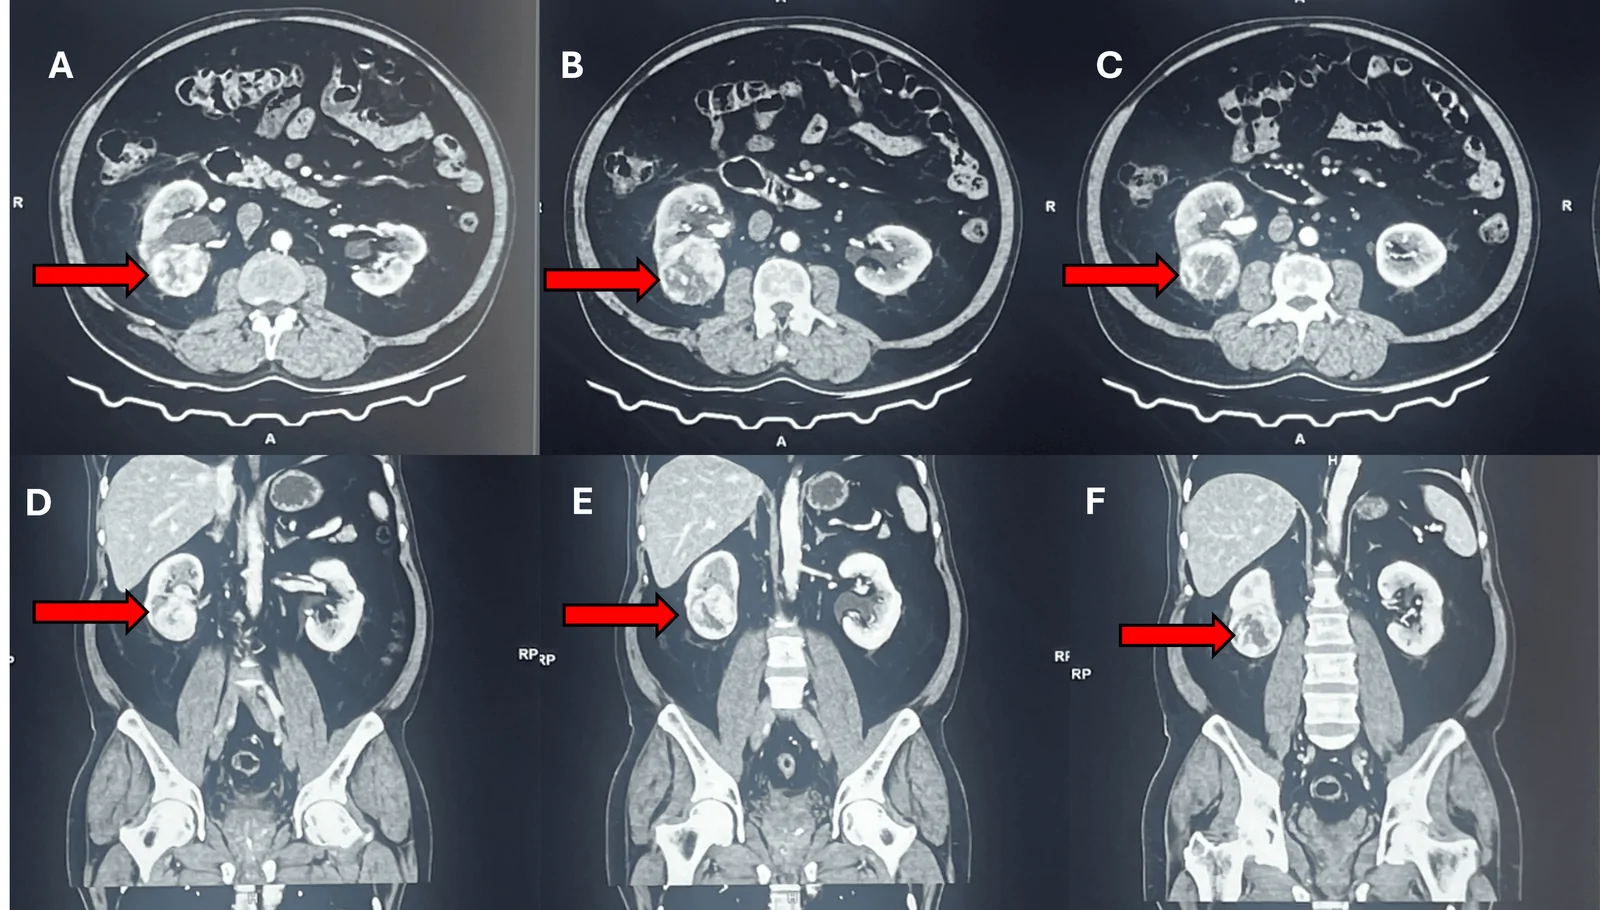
Contrast-enhanced computed tomography of the abdomen showing renal mass. (A, B, C) Axial section. (D, E, F) Coronal sections. Orange arrows indicate a well-defined, heterogeneously enhancing soft-tissue density mass lesion measuring 5.0 x 4.7 x 4.0 cm in the lower pole of the right kidney, along the postero-inferior aspect, with a predominantly exophytic component and no evidence of calcification or fat density lesions.
